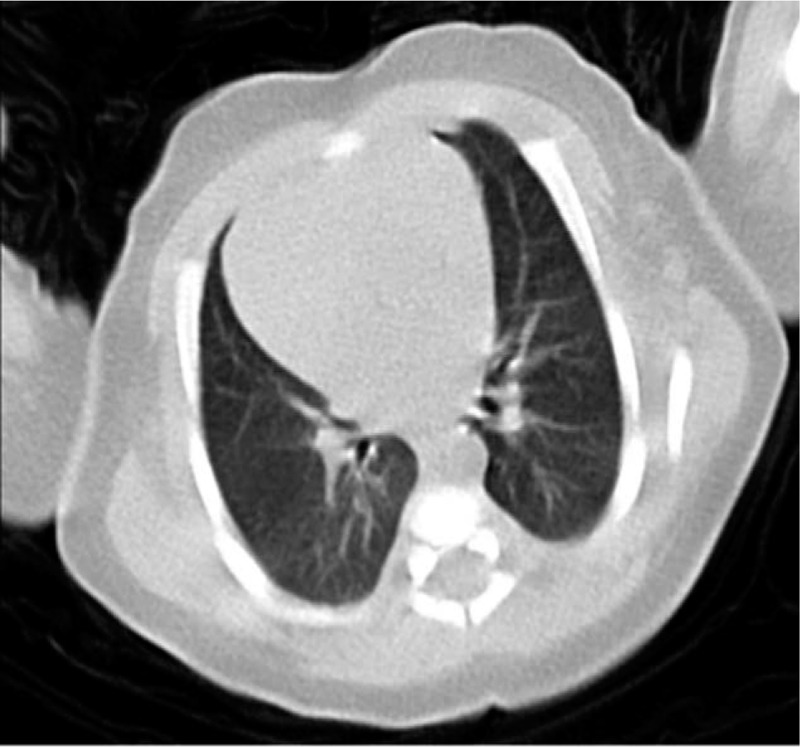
Chest computed tomographic images of the infant infected with SARS-CoV-2. No thoracic abnormalities were noted on February 12, 2020 (Illness Day 3). SARS-CoV-2 = severe acute respiratory syndrome coronavirus 2.
